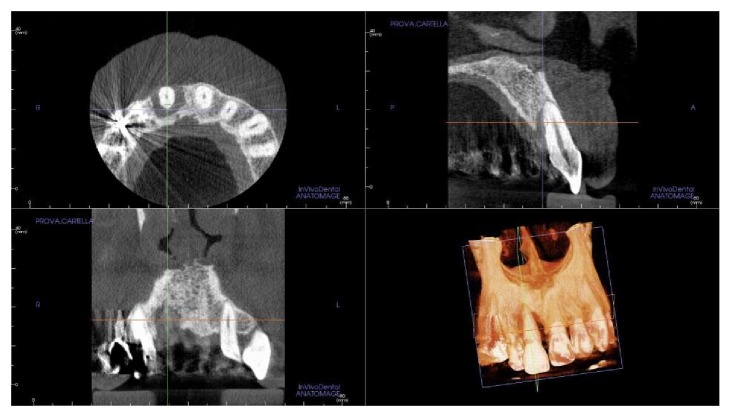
The small field-of-view (FOV) cone-beam computed tomography (CBCT) with three-dimensional (3D) reconstruction by means of an implant navigation software (Invivo Dental 5, Anatomage, San Jose, CA, USA) confirmed the presence of an advanced, localized bone resorption affecting the right-maxillary central incisor.
